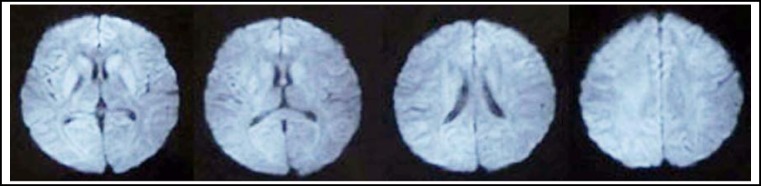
DWI (2013-06-07) showed symmetric abnormal signals in bilateral caudate nucleus and putamen
